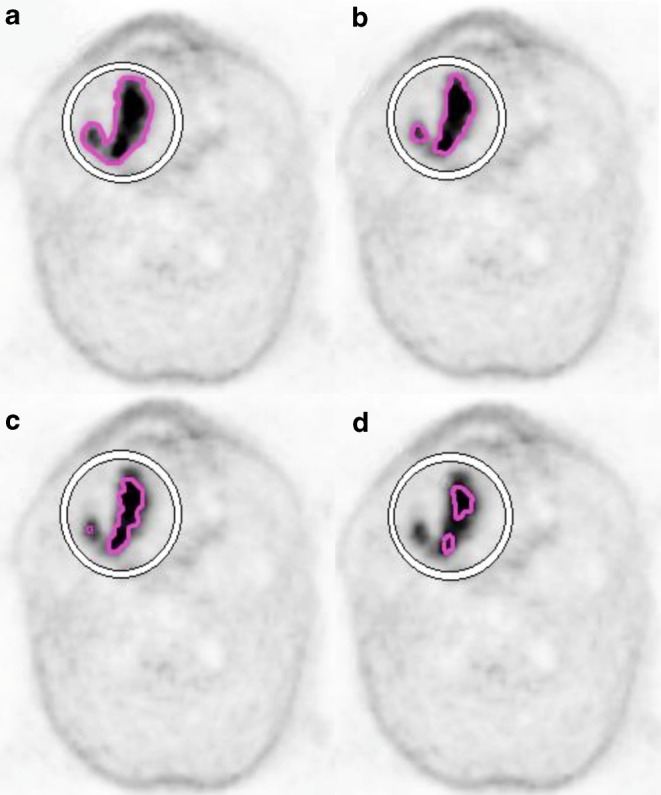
Comparison of primary tumor (GTV, gross tumor volume) delineation using automatic fixed threshold method in patient with squamous cell carcinoma of the tongue (T3N2bM0): a GTV obtained with threshold of 20% of SUV_max_ (maximum standardized uptake value), b threshold of 30% of SUV_max_, c threshold of 40% of SUV_max_, d threshold of 50% of SUV_max_
